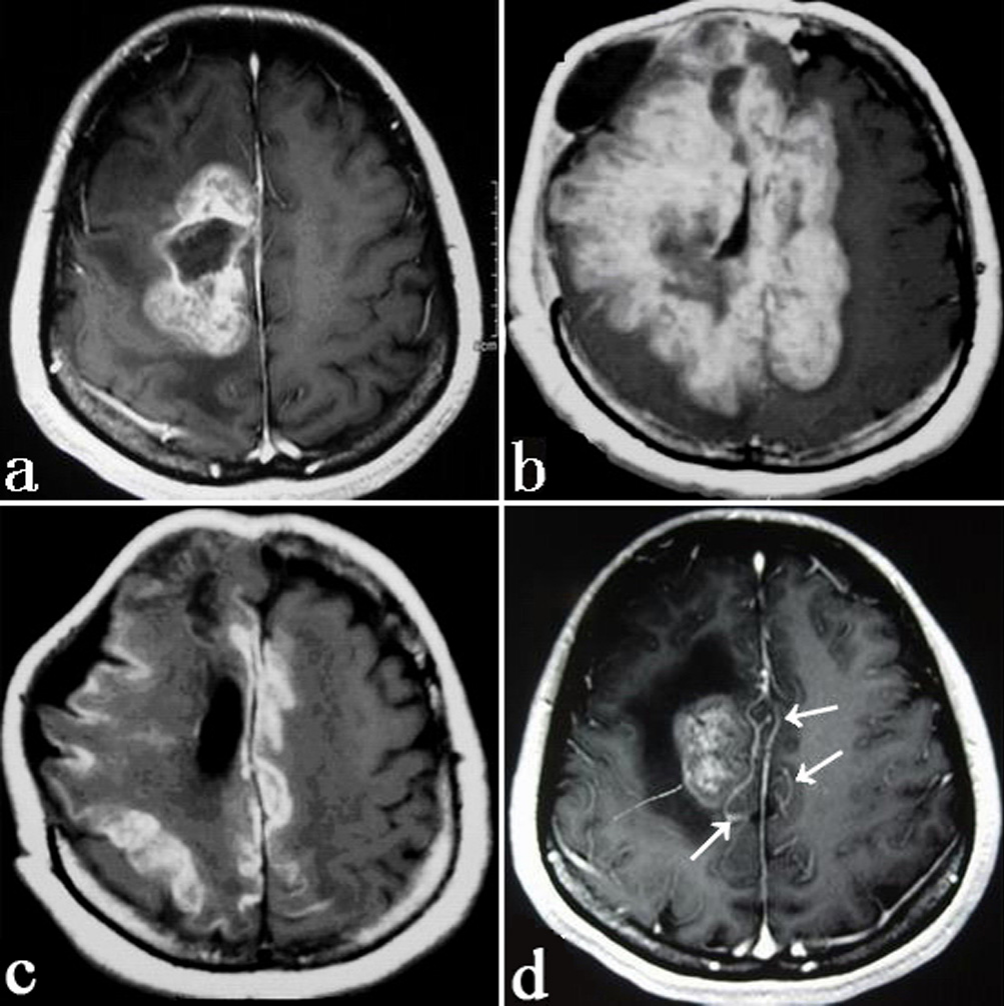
Axial contrast-enhanced MR image showing a partial resection of the lesion. (a) T1-weighted image showing the newly developed hyper-intensity diffusing to the opposite side (b). Great improvement after the treatment (c). T1-weighted MR images with contrast showing some abnormally enhanced vessels (arrows) (d).
